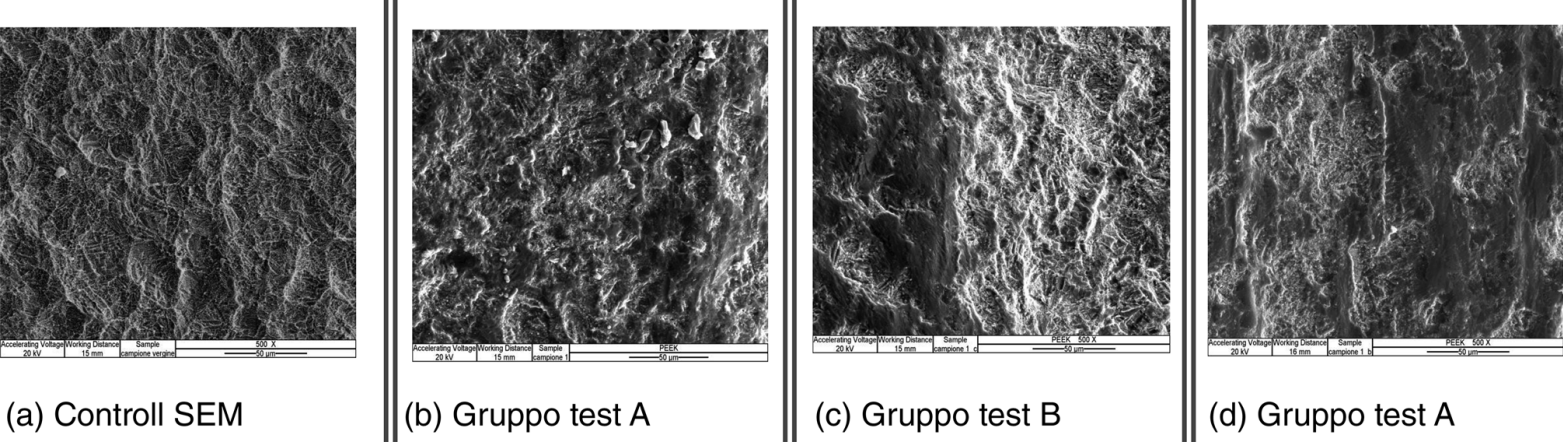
In the ×500 scans instrumented with a peek insert (b–d), compared to the virgin control (a), the areas of marked sanding of the surface with a vertical and horizontal trend, due to the action of the Peek tip mounted on the ultrasonic handpiece, remain more visible between the normal implant texture of the implant. Gruppo test (B) versus Controllo SEM a ×500
